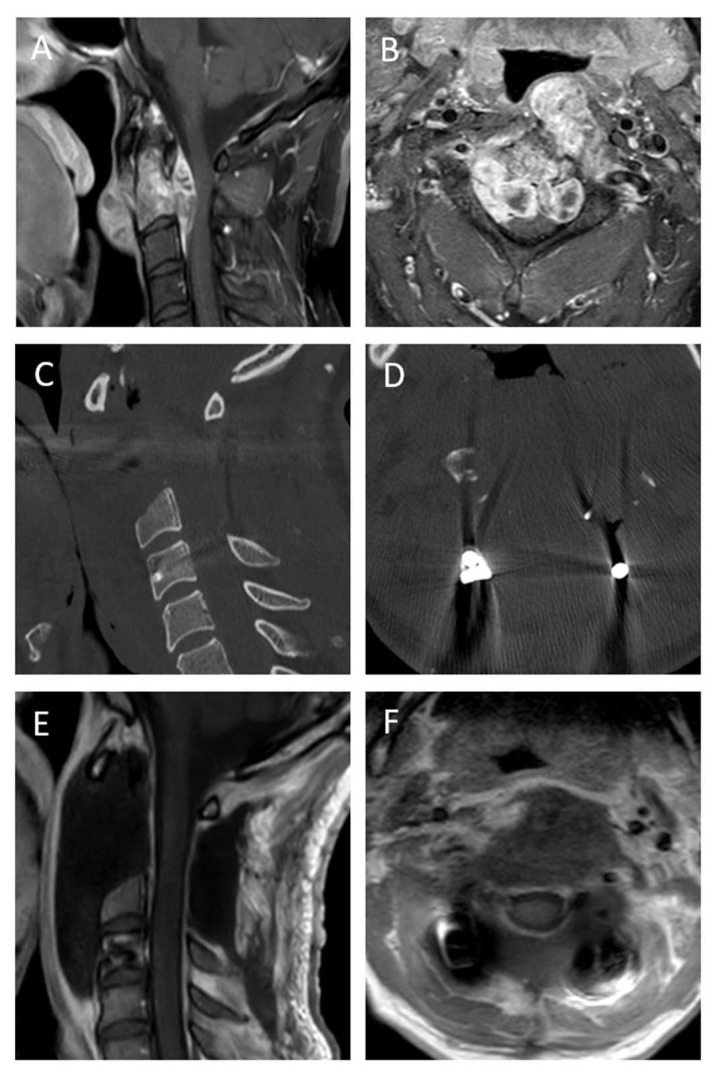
Pre- and postoperative imaging of a case of a chordoma of C2 with significant spinal cord compression. (A,B): Sagittal and axial T1 contrast-enhanced MRI imaging showing the extent of the tumor with ventral compression of the spinal cord, (C,D): Postoperative CT imaging after dorsal instrumentation of C1–4 with titanium lateral mass and pedicle screws and dorsal release and consecutive EI resection, (E,F): Postoperative T1 + contrast MRI imaging showing complete resection of the tumor.
